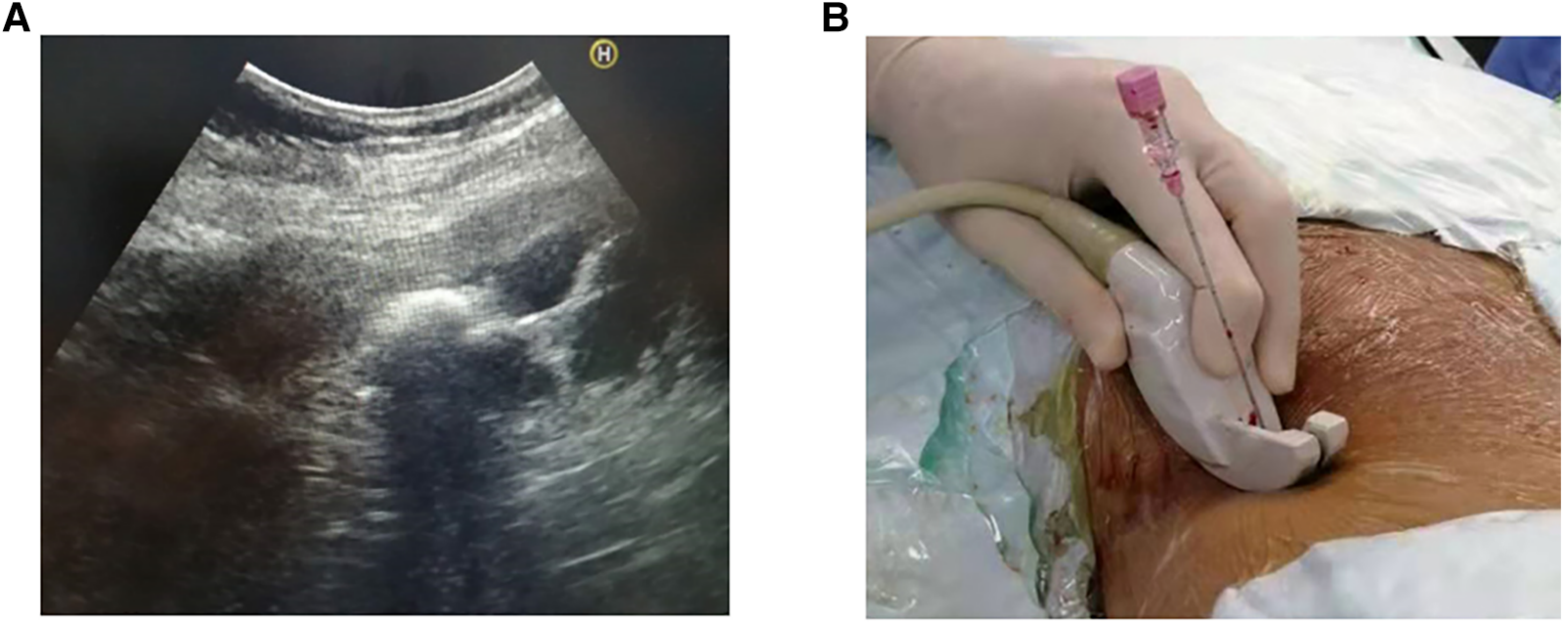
Ultrasonography guided localization and puncture.
